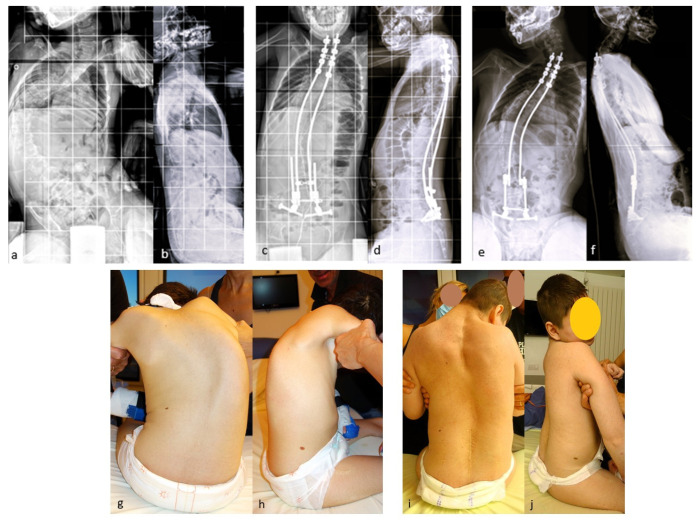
(a,b) Initial radiographs of a 12-year-old CP boy; (c,d) Immediate postoperative radiographs; (e,f) 5 year postoperative radiographs showing the expansion of the rods; (g–j) initial and 5 year postoperative clinical pictures.
